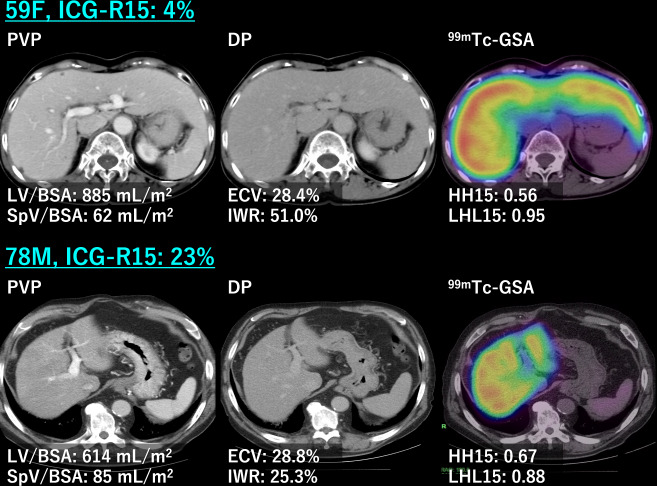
Axial contrast-enhanced CT images during PVP (left column) and DP (middle column) and fused ^99m^Tc-GSA SPECT/CT images (right column). The upper row shows a 59-year-old woman with normal liver function according to ICG-R15 (4%) and ^99m^Tc-GSA. The lower row shows a 78-year-old man with severe liver dysfunction according to ICG-R15 (23%) and ^99m^Tc-GSA. LV/BSA was higher and SpV/BSA was lower in the high-functioning patient. ECV did not reflect any difference between the patients. The IWR reflected ICG-R15 and ^99m^Tc-GSA well, being 51% in the normal functioning patient and 25% in the poor
